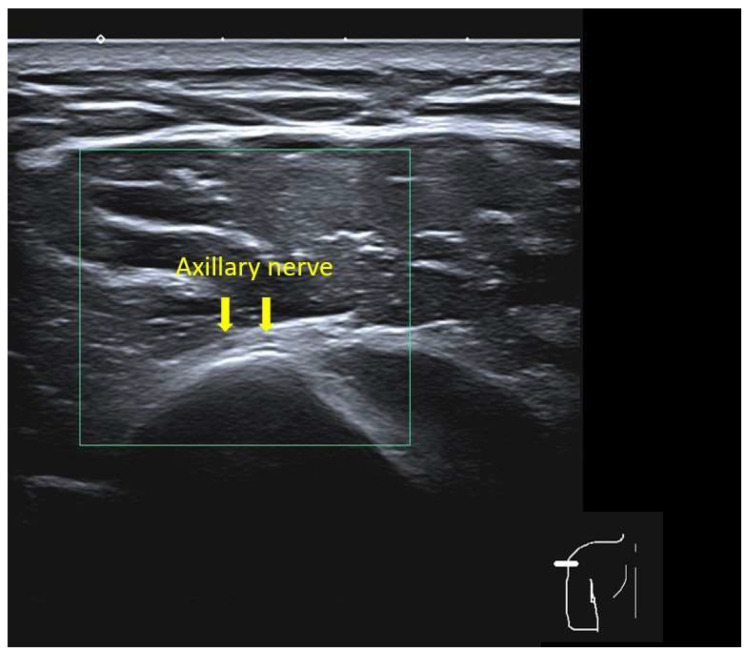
Ultrasonographic image demonstrating the axillary nerve (yellow arrow).
